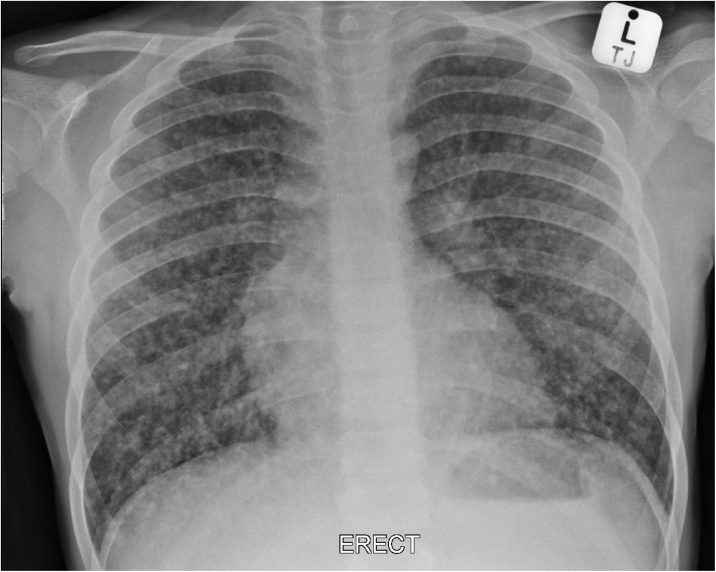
Chest roentgenogram shows bilateral diffuse micronodular opacities.
